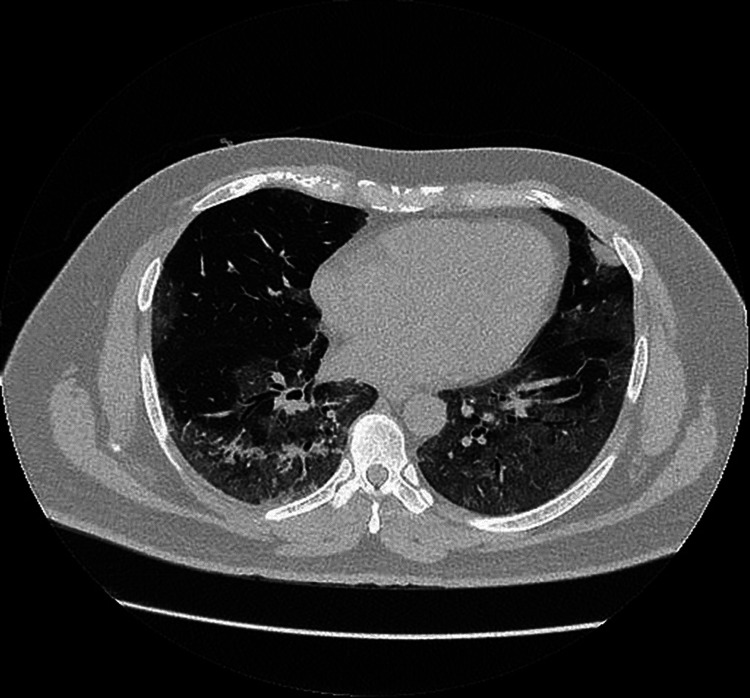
Computed tomography (CT) chest  demonstrated bilateral extensive ground-glass opacification at the peripheral lung zones
